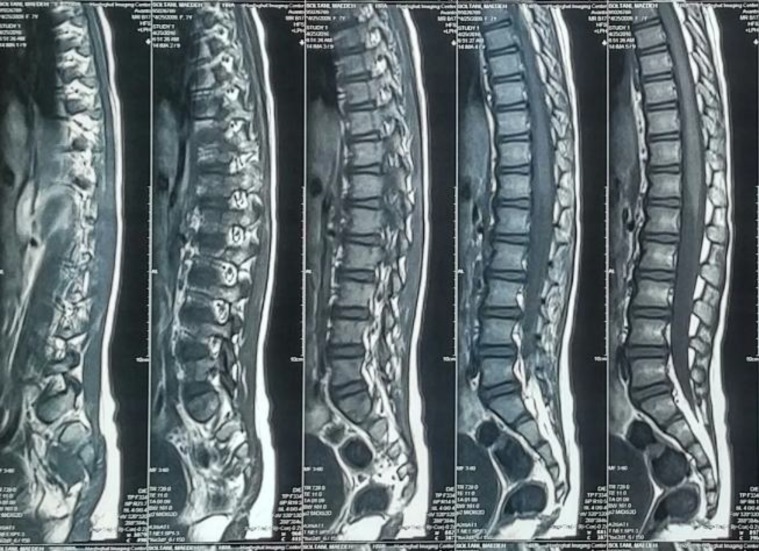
Sagital T1 weighted MRI showed spinal subdural hematoma extended from T2 to T6 (Arrow)
